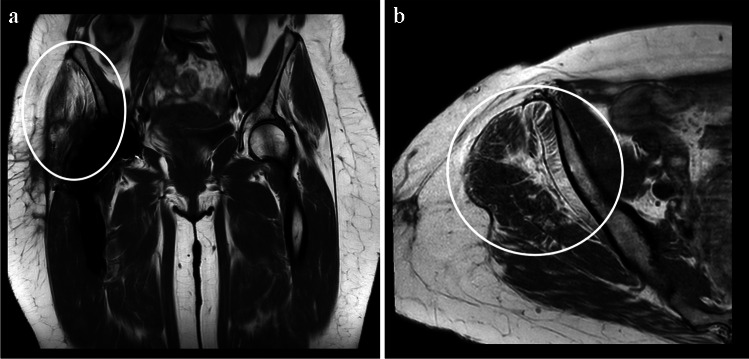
MRI showing fatty atrophy of the right-side gluteus minimus and medius. T1-weighted sequences in a the coronal and b the axial plane 3 months after THA. The findings indicate Goutallier 3 fatty atrophy
